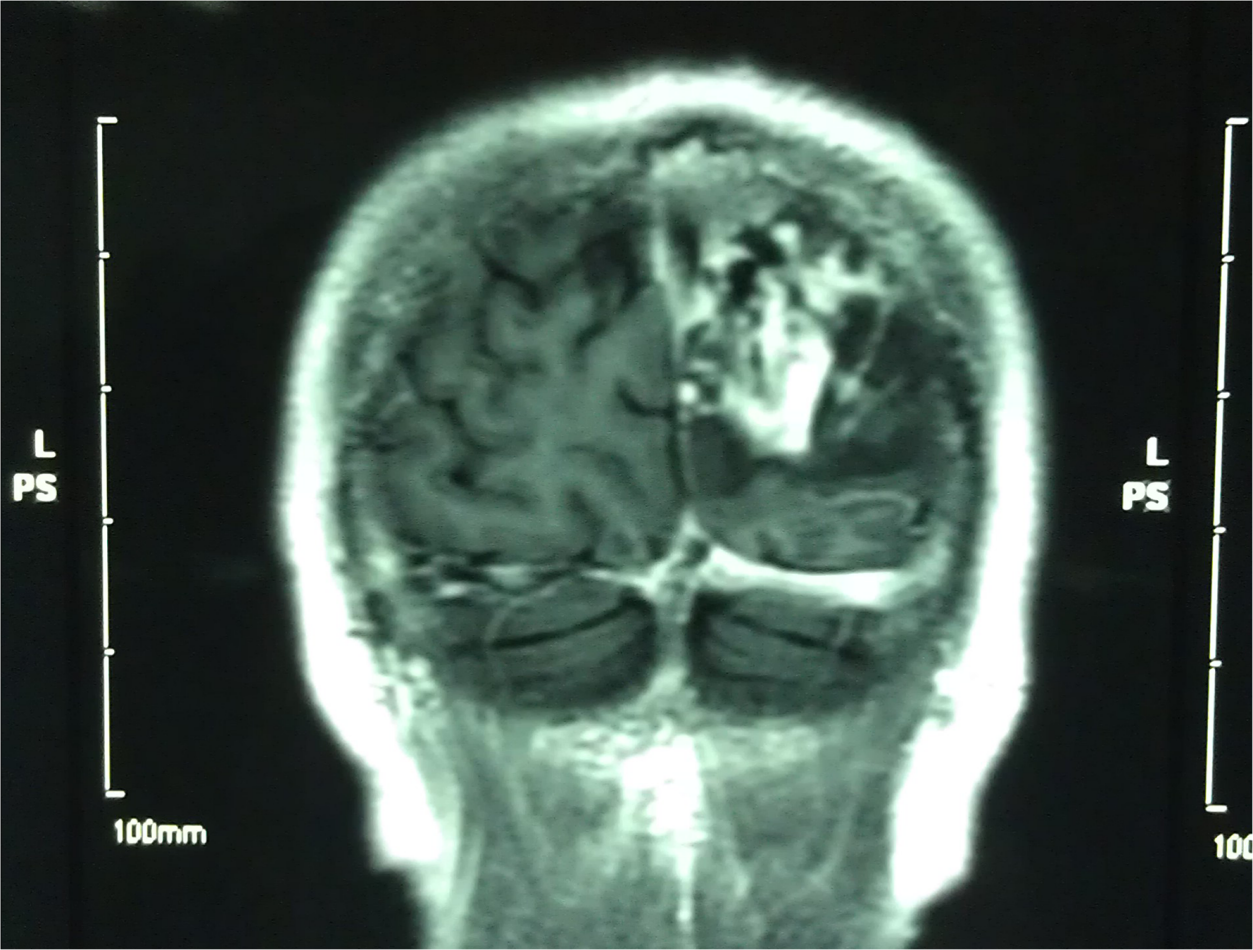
MRI of the brain before treatment. MRI of the brain before treatment shows an occupied lesion in the left occipital region.
